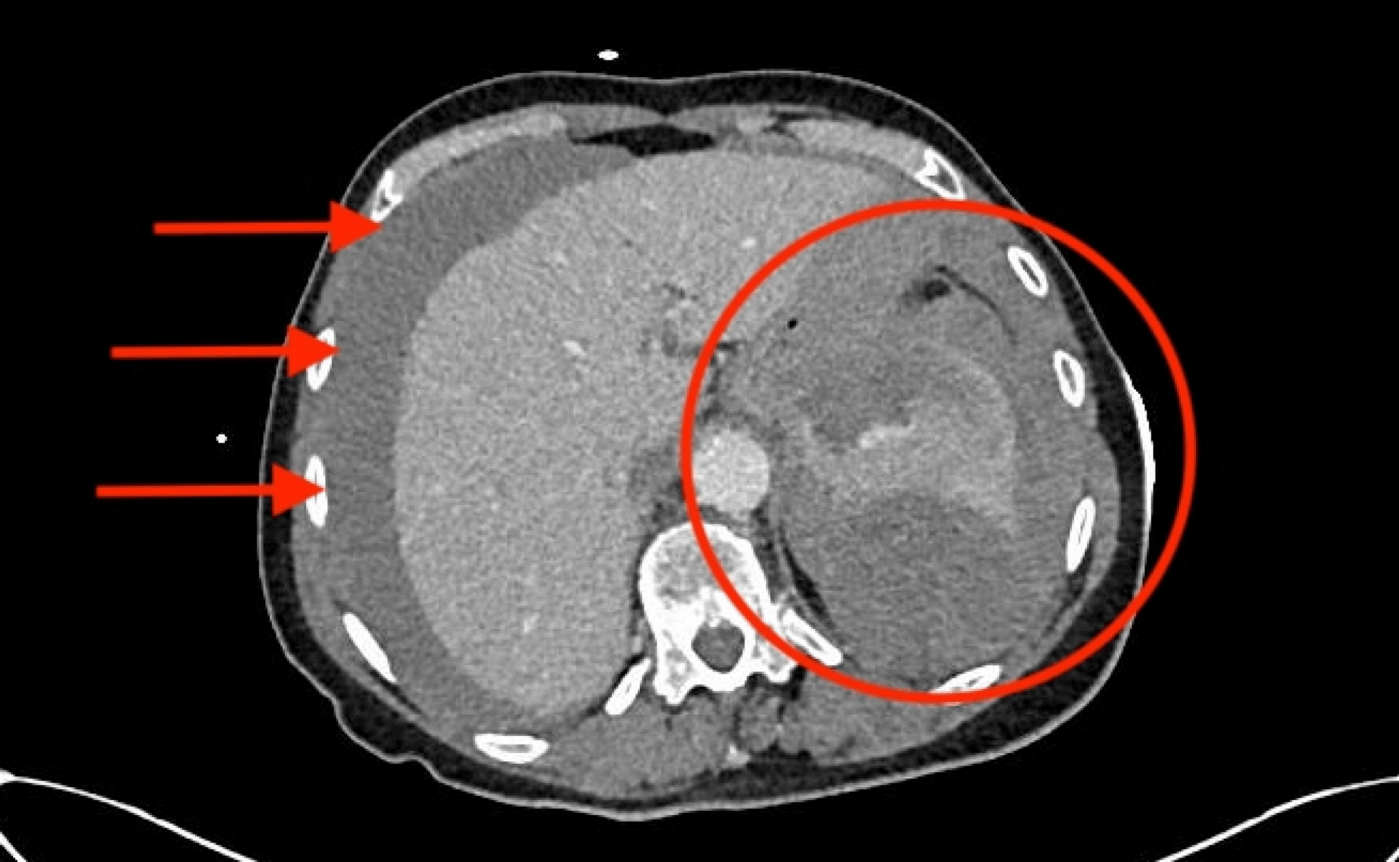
Axial CT abdomen/pelvis at a mid-splenic level showing hemoperitoneum and active splenic bleeding. Red arrows highlight contrast extravasation. Red circle denotes the main region of hemorrhage.
